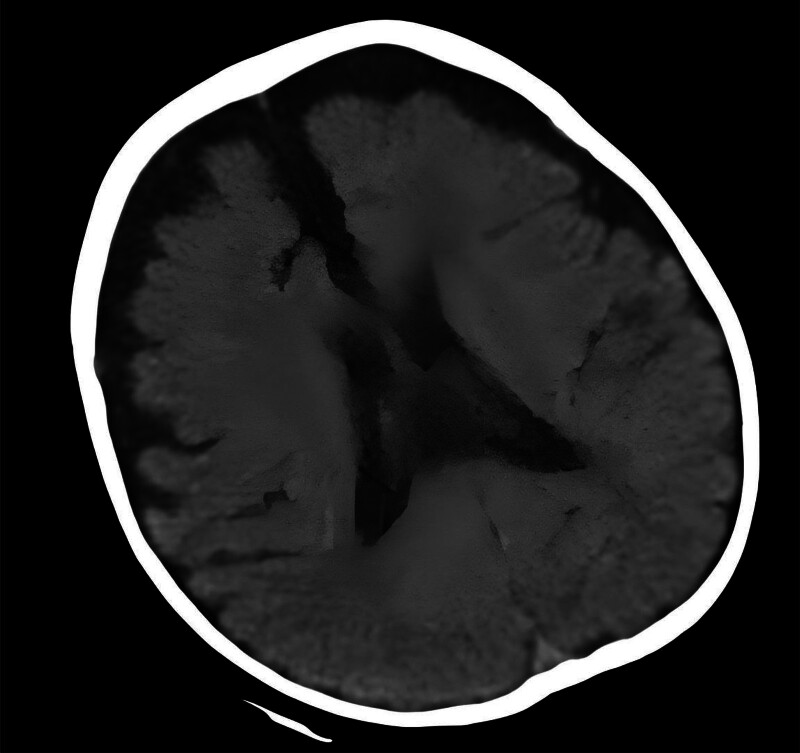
Craniocerebral HRCT showed the bilateral frontotemporal subarachnoid space was enlarged, the brain sulci and brain fissure were widened and deepened, external hydrocephalus. HRCT = high-resolution computed tomography.
